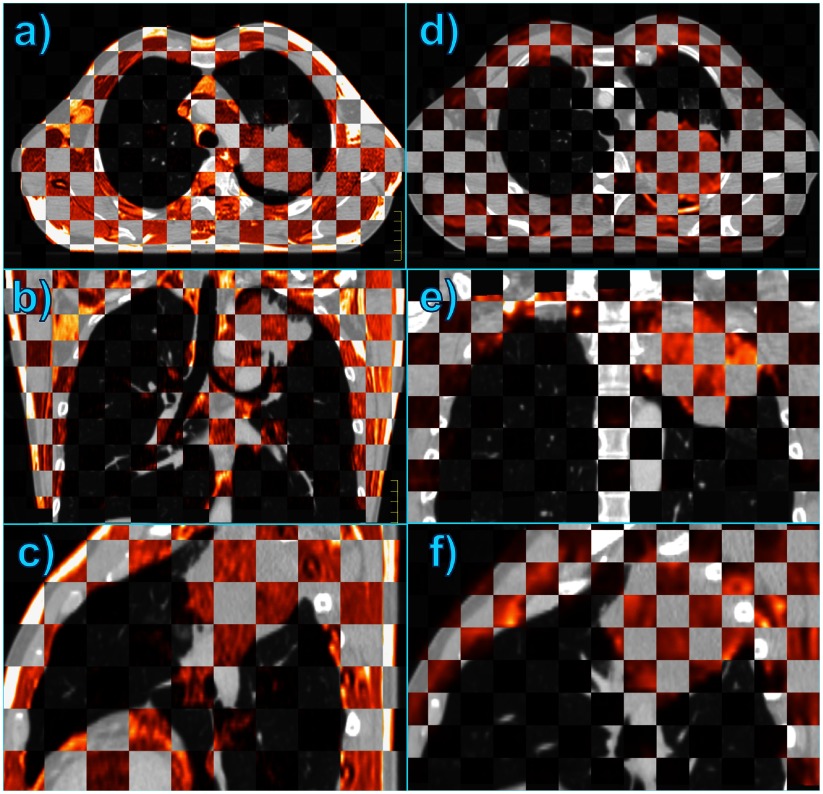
(*a*) Checkerboard view of the Pinnacle^3^ registration of an axial CT image (grey) with an axial 3D T1-w VIBE image (orange) of a NSCLC patient in ABC-controlled breath holding with the same settings and positioning. (*b*) Coronal and (*c*) sagittal reconstruction of the CT-VIBE fusion. (*d*) A more superior transversal slice of the CT image of the same patient (grey) fused with a *b* = 200 s mm^−2^ DW-EPI1 (orange) acquired under the same ABC conditions. (*e*) and (*f*) denote the coronal and sagittal reconstruction of the CT-EPI fusion. All of these images demonstrate the good inter-modality registration achievable with the ABC system.
